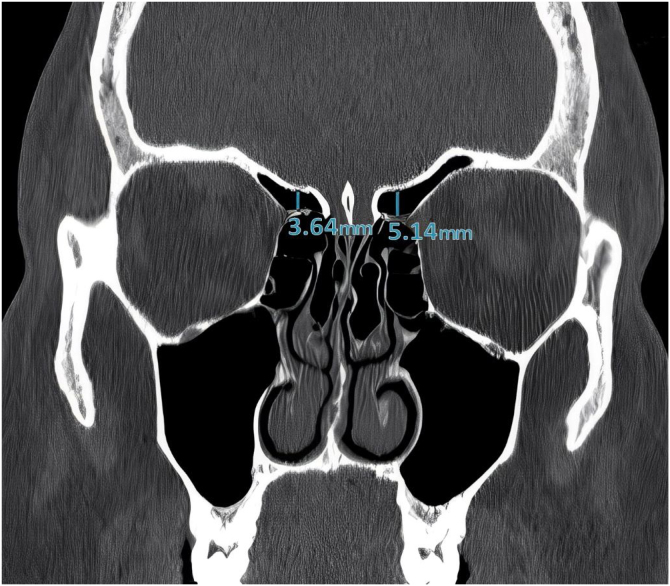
Computed tomography showing lateral distance variation.
